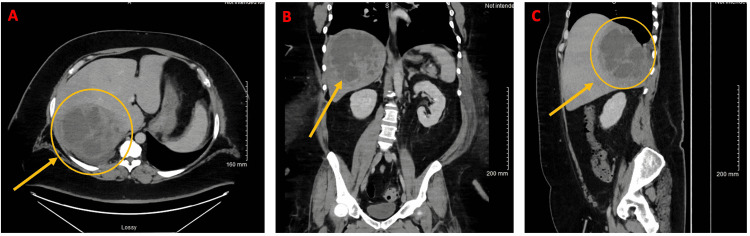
CT of the abdomen/pelvis with contrast on hospital day five (first admission) (A) Axial view of liver abscess. (B) Coronal view. (C) Sagittal view.
